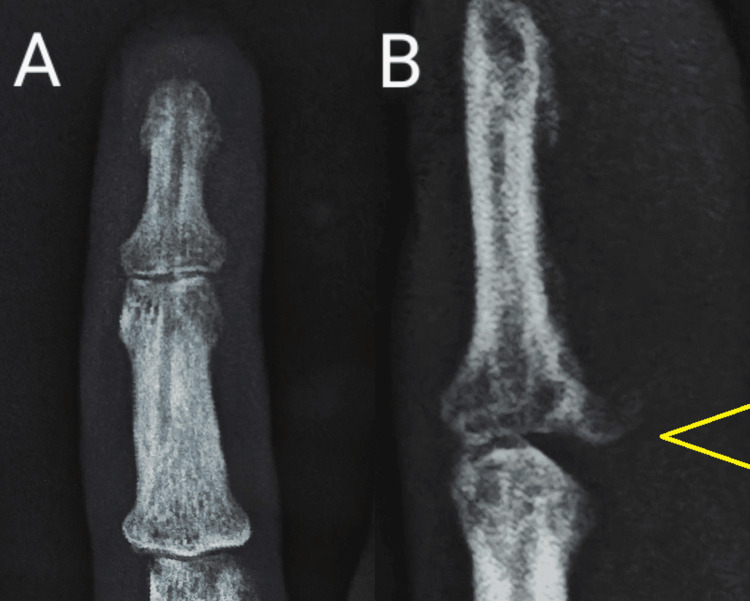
Follow-up radiograph after K-wire removal (A) AP view. (B) Lateral view.
